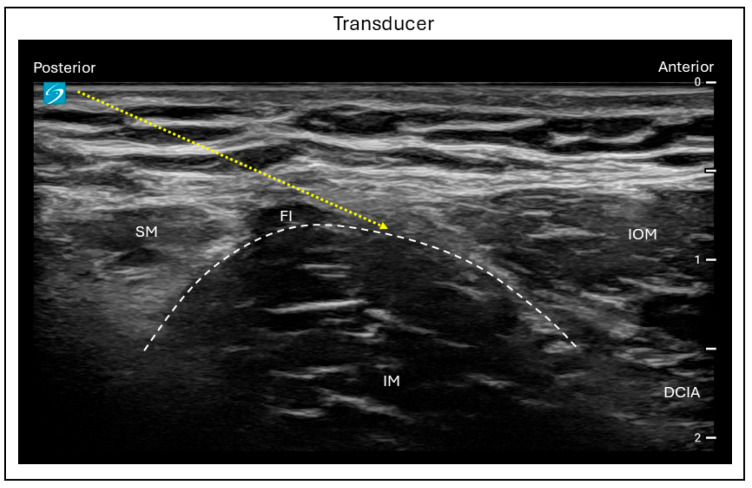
Ultrasound image demonstrating the in-plane suprainguinal fascia iliaca block technique. FI = Fascia iliaca; SM = Sartorius muscle; IM = Iliacus muscle; IOM = Internal oblique muscle; DCIA = Deep circumflex iliac artery; Dashed line = Needle trajectory.
